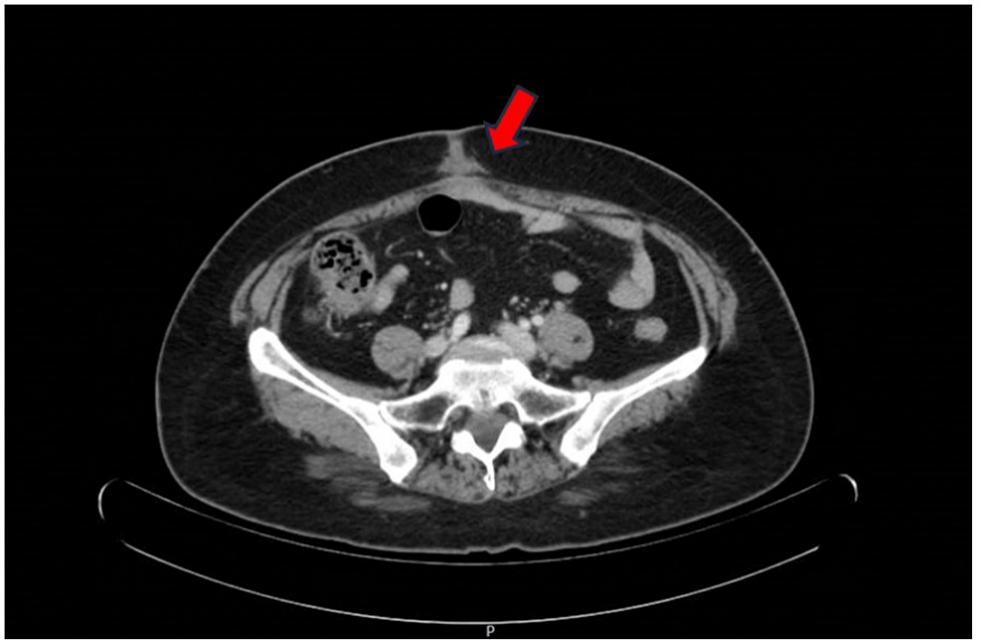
CT scan of the abdomen showing haziness of subcutaneous fat in the periumbilical area, probably sequela of umbilical hernia repair. CT: Computed tomography
